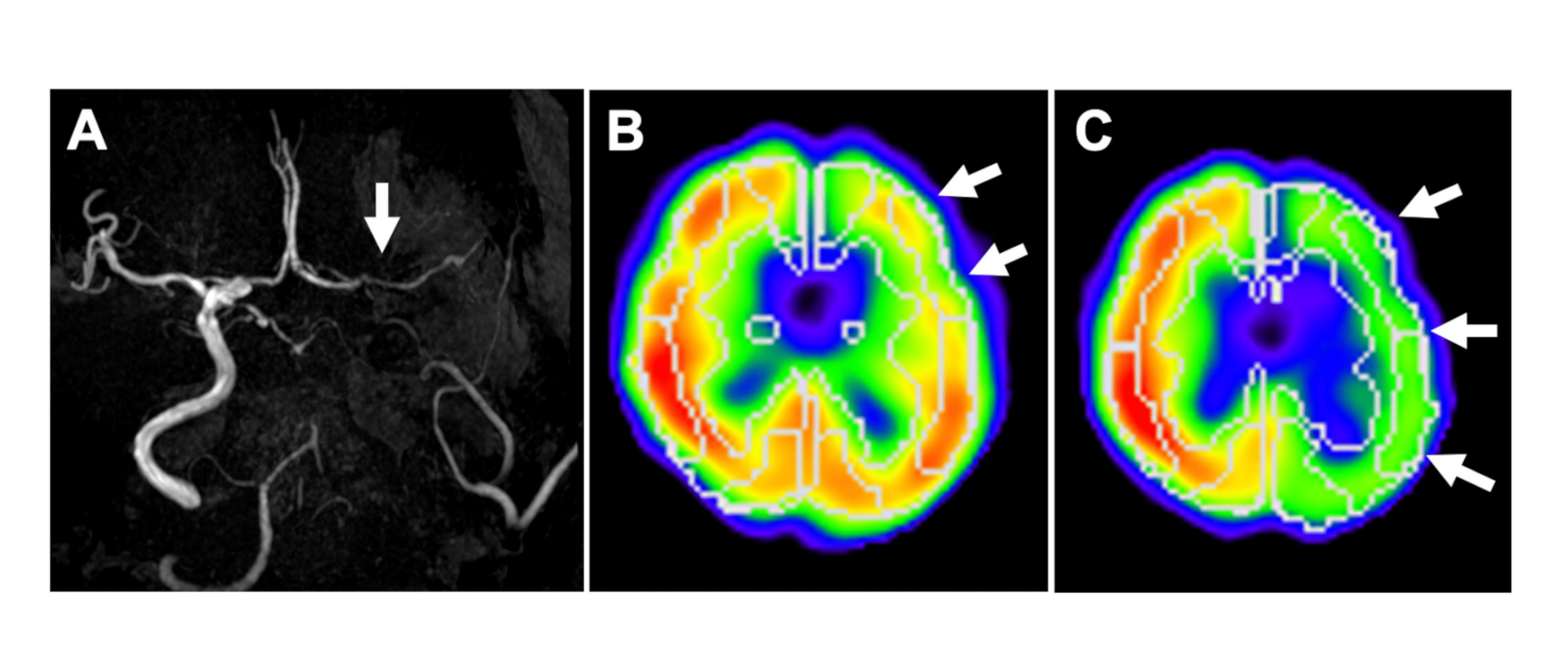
Preoperative magnetic resonance angiography and single-photon emission computed tomography (A) Preoperative magnetic resonance angiography showing the left internal carotid artery (ICA) occlusion (white arrow). (B) Preoperative single-photon emission computed tomography (SPECT) with N-isopropyl-p-[^123^I]iodoamphetamine (^123^I-IMP) was performed to assess cerebral blood flow (CBF) and cerebrovascular reactivity (CVR) to acetazolamide, showing cerebral hypoperfusion (white arrows) and (C) decreased CVR in the left middle cerebral artery (MCA) (white arrows).
